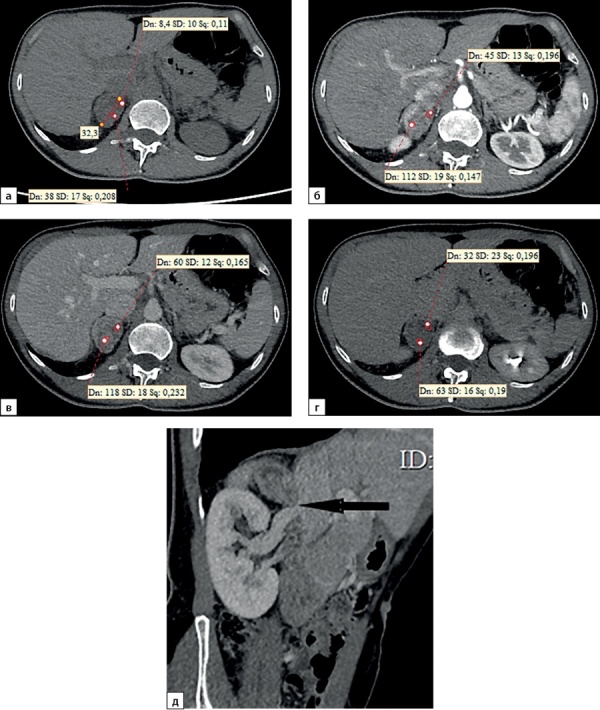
Рисунок 4. Компьютерные томограммы пациентки Ч., 42 лет с небольшой адренокортикальной карциномой (32х28 мм), с гетерогенной опухолевой тканью, распространяющейся до ворот почки: а — изображение в аксиальной плоскости, нативная фаза сканирования, плотность от +8,4 до +38HU; б — артериальная фаза постконтрастного сканирования; в — венозная фаза; г — фаза отсроченного сканирования, д — изображение реформатированное в косой сагиттальной плоскости, венозная фаза сканирования, образование локально деформирует почечную вену (стрелка). Коэффициент абсолютного вымывания контрастного препарата составил 56%, относительного — 36%.Figure 4. Computed tomography of patient Ch., 42 years old, with a small adrenocortical carcinoma (32x28 mm), with heterogeneous tumor tissue extending to the hilum of the kidney: a — image in the axial plane, native scanning phase, density from +8.4 to +38HU ; b — arterial phase of post-contrast scanning; c — venous phase; d — phase of delayed scanning, e — image reformatted in the oblique sagittal plane, venous phase of scanning, the mass locally deforms the renal vein (arrow). The coefficient of absolute washout of the contrast agent was 56%, relative — 36%.
